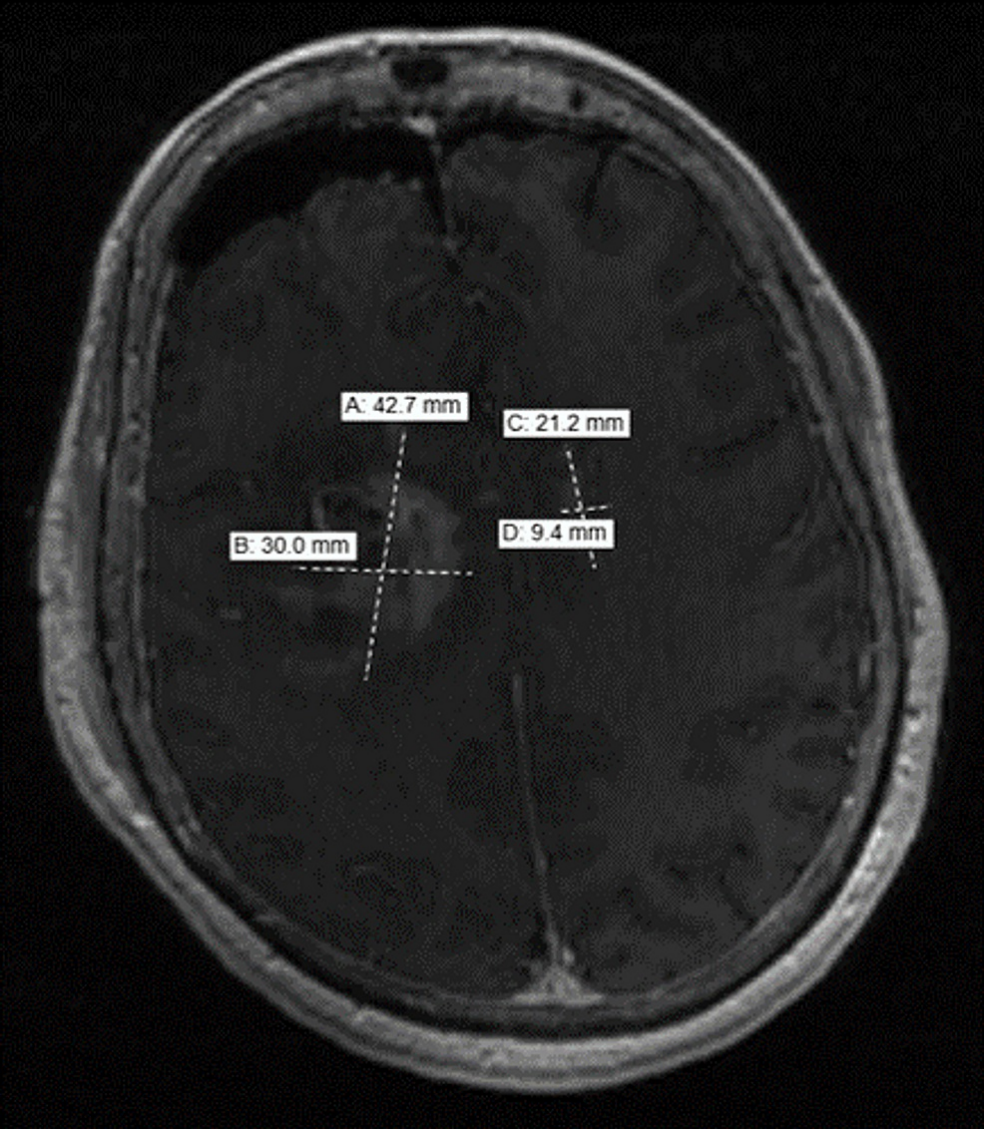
Post-op MRI showing debulked tumor with persistent surrounding edema.
